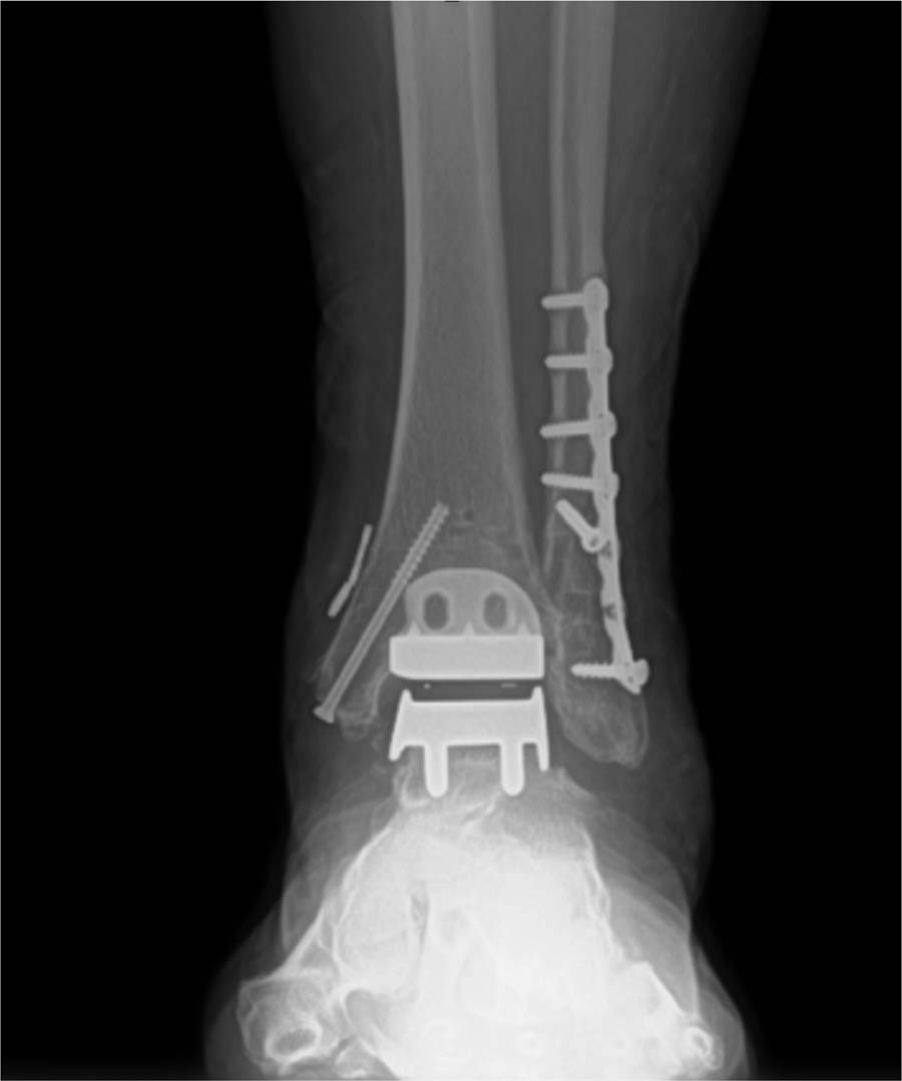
Post-operative radiograph without any sign of gutter impingement or ectopic bone formation.
